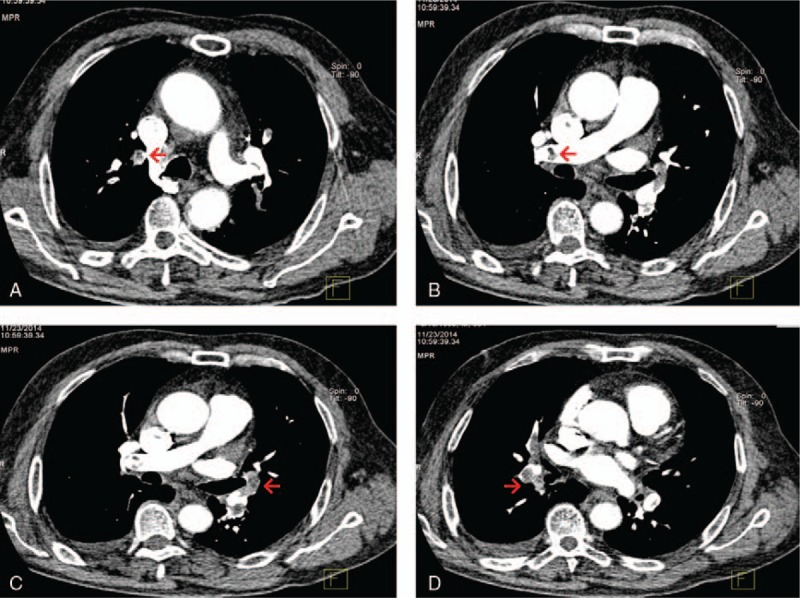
Computed tomography image showing pulmonary embolism (red arrows). A-D, showing PE in different scanning levels.
